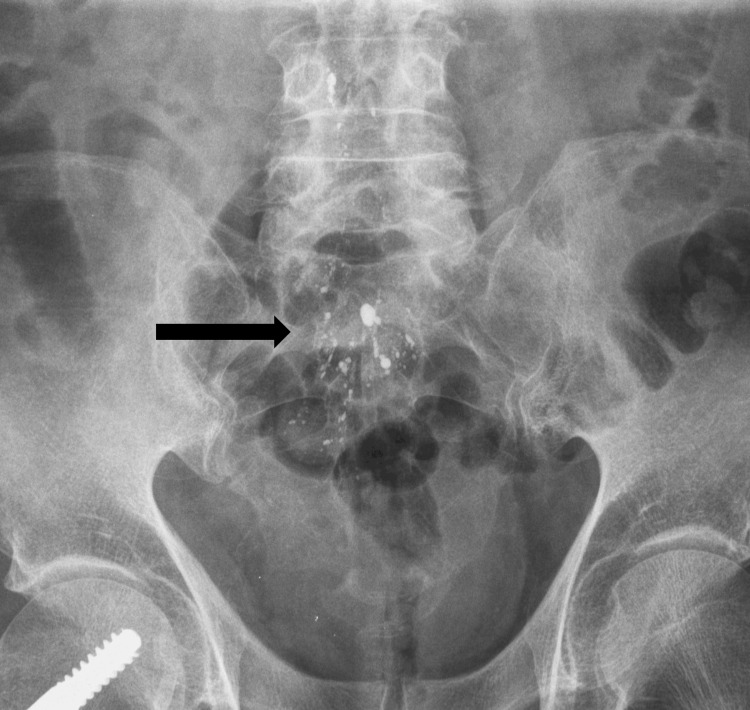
Antero-posterior lumbar X-ray. Antero-posterior lumbar X-ray showing multiple opacities around the cauda equina (arrow).
